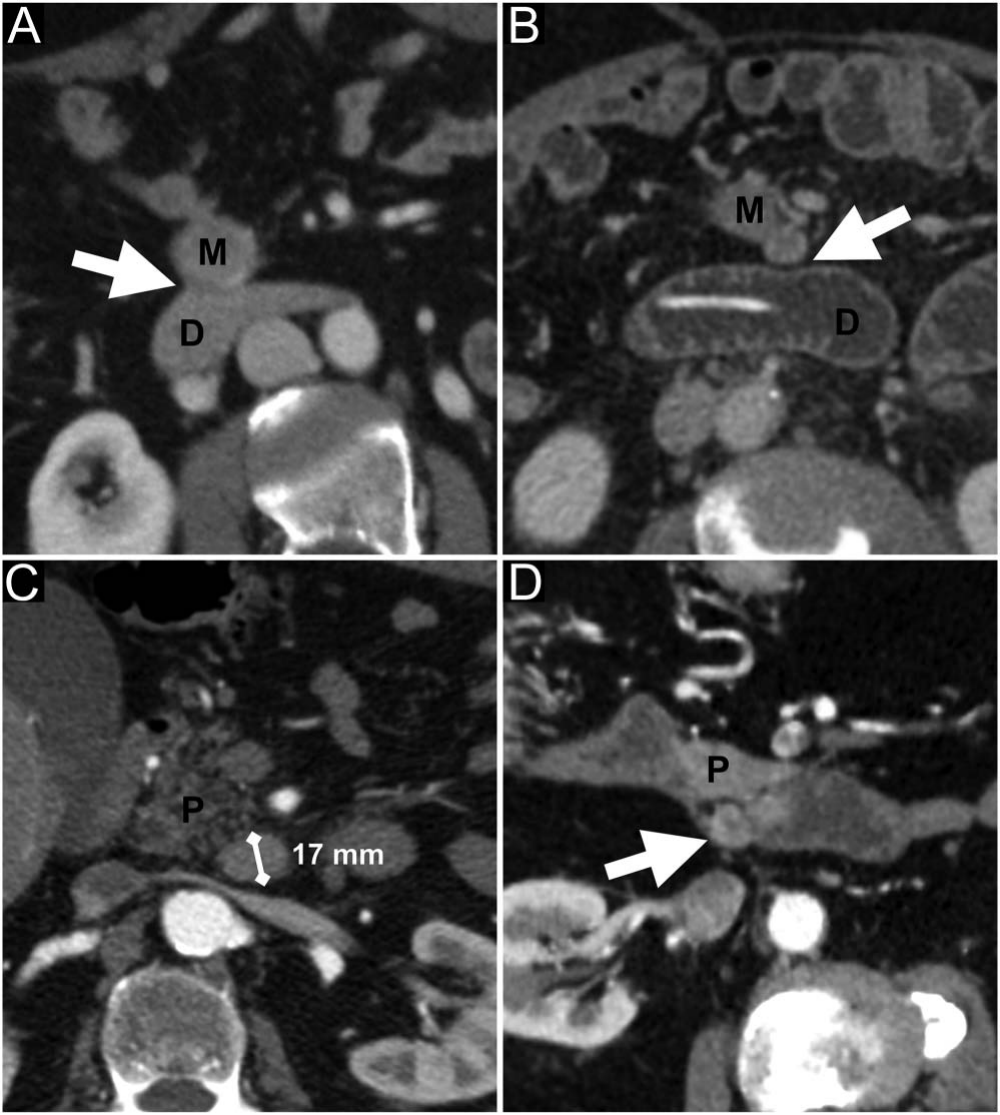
Duodenal and retropancreatic analysis on CT. M, mesenteric mass; D, duodenum; and P, pancreas. (A) The patient is a 55-year-old woman. Portal phase – axial. The white arrow shows the loss of the fat plane between the mesenteric mass (M) and the duodenum (D). (B) The patient is a 64-year-old man. Portal phase – axial – enteroclysis. The white arrow shows the persisting fatty plane between the mesenteric mass (M) and the duodenum (D). (C) The patient is a 76-year-old man. Arterial phase – axial. Retropancreatic lymphadenopathy retained as pathological because of the small axis >10 mm and the enhancement on arterial phase. (D) The patient is a 57-year-old man. Arterial phase – axial. Retropancreatic lymphadenopathy (white arrow) retained as pathological because of the enhancement on arterial phase and the round shape.
